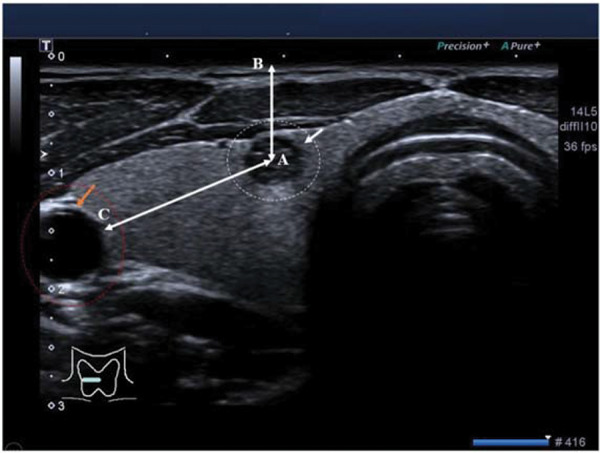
Grayscale ultrasound image of the right thyroid lobe of a 36-year-old female patient. The nodule was 3 mmin size. The white circle and white arrow indicate a suspicious thyroid nodule. The saffron circle and saffron arrow indicate the carotid artery. AB: the depth of the nodule and AC: the distance of the nodule from the carotid artery.
